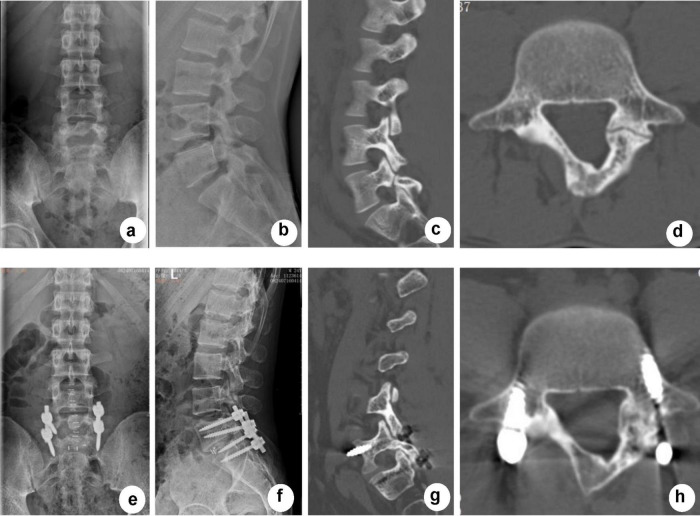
Typical case of SSLIF. A 35-year-old female patient who had suffered from low back pain for 3 years underwent SSLIF surgery. The pain was significantly reduced 1 year after surgery without ASD, screw loosening or rod fracture. (a,b) Anteroposterior and lateral X-ray CT before surgery showed L5 spondylolysis. (c,d) CT before surgery showed bilateral L5 spondylolysis. (e,f) Anteroposterior and lateral X-ray after surgery showed that SSLIF provided strong fixation and that the cage was in the proper position. (g,h) CT showed that pedicle screws in L5 and S1 were in position without loosening 1 year after surgery.
